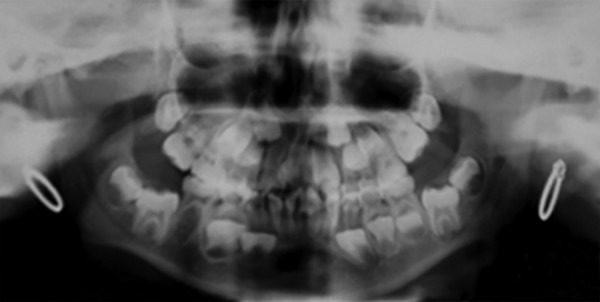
Orthopantomography revealing erupting 11, 22, 36, 46
